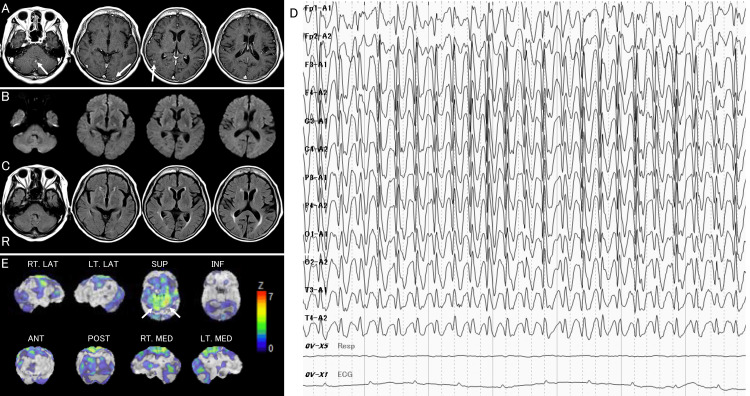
Neuroimaging of Case 2 at the first attack A: T1-weighted imaging four months before the first attack revealing well-enhanced lesions in the cerebellar vermis and bilateral occipital lobes, indicating metastatic tumors (arrows). B: Diffusion-weighted imaging 22 hours after onset, revealing no new lesions. C: Fluid-attenuated inversion recovery imaging at 22 hours after onset showing no lesions, including metastatic lesions, which were observed four months ago. D: Electroencephalography at 22 hours after onset, revealing spike and wave complex in the bilateral whole brain. E: Single-photon emission computed tomography on Day 5, revealing a high-uptake lesion in the bilateral superior and inferior parietal lobules (arrows).
